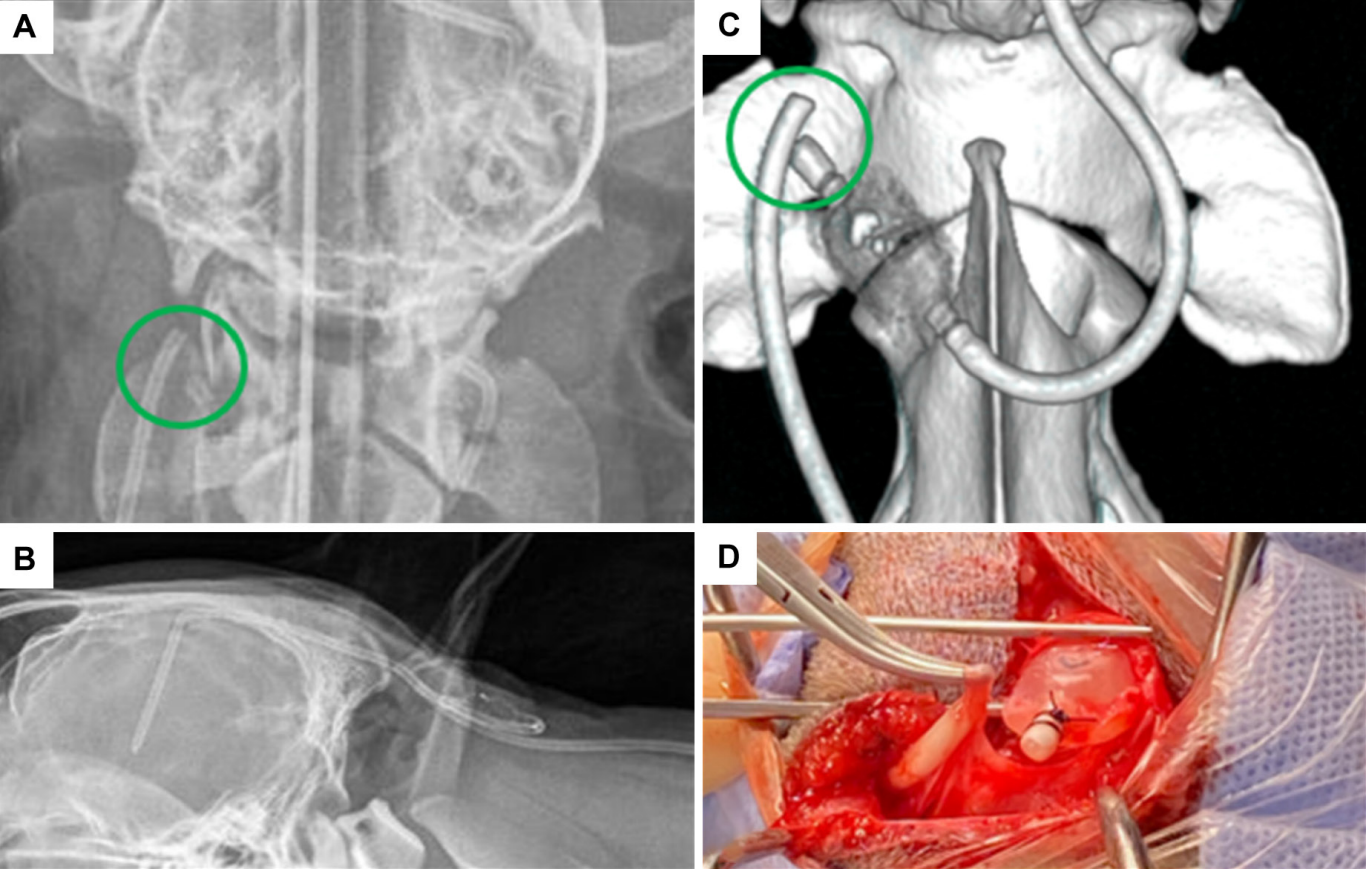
(A) Dorsoventral radiographic image of atlantooccipital region reveals a discontinuation in the ventriculoperitoneal shunt (VPS) catheter (green circle) situated over the right transverse process of C2. (B) Right lateral radiograph of the same anatomical location demonstrates poor visualisation of the shunt rupture. (C) Three‐dimensional computed tomography reconstruction image of the cranial cervical area provides clearer visualisation of the catheter rupture (green circle). (D) Intraoperative image of the patient verifies the presence of catheter rupture and intact sutures connecting the cranial end of the distal catheter to the VPS valve.
